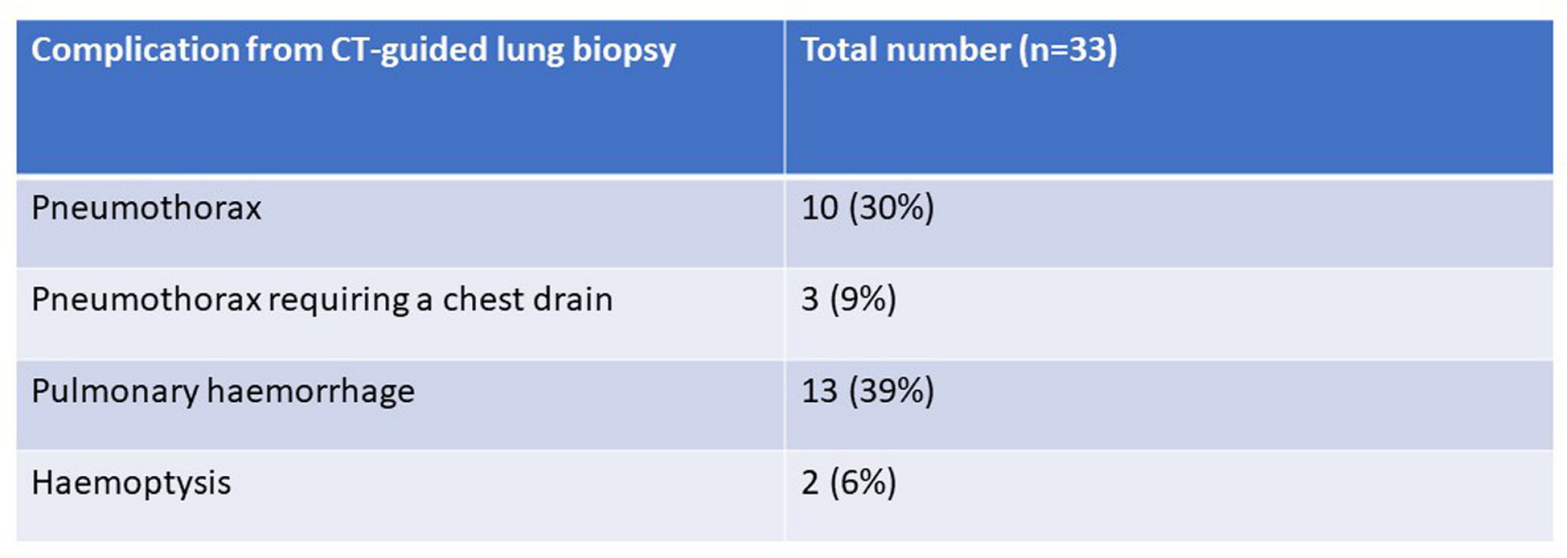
Complications from CT-guided lung biopsies
